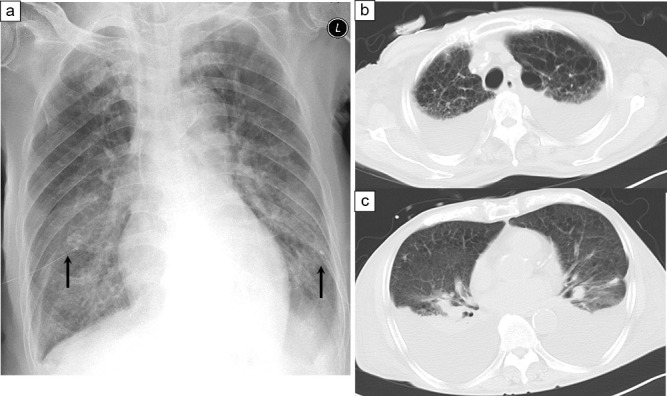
Imaging study on admission. a: Chest X-ray (supine position); b, c: Chest CT. a: Pleural effusion and thoracic drainage tubes were placed on both thoracic cavities (black allow). b, c: Moderate amounts of pleural effusion were observed on both sides.
